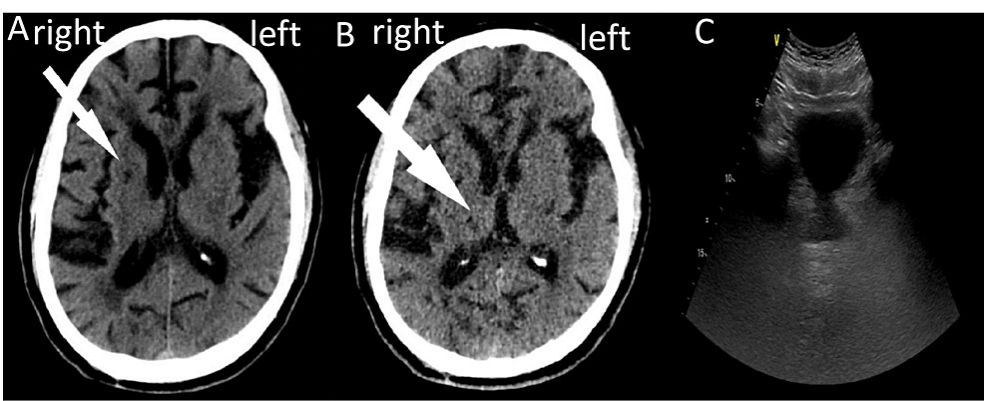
Computed tomography scan and prostate ultrasound A: right basal lacunar infarction, B: right thalamus lacunar infarction, C: normal prostatic ultrasound findings
